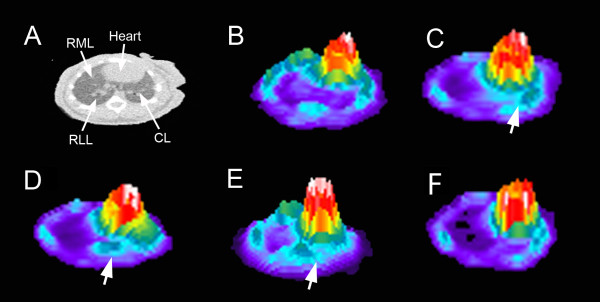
Intensity plots of FDG uptake in the cardiac lobe at various times after pneumonectomy. (A) A CT image of the transverse plane of the post-pneumonectomy lung identifying the location of the heart, right middle lobe (RML), right lower lobe (RLL), and cardiac lobe (CL). FDG-PET intensity profiles of a similar transverse plane on the day of surgery (B) and days 3 (C), 7 (D), 14 (E), and 21 (F) after pneumonectomy. Apparently increased uptake in the cardiac lobe is denoted (large arrow). Representative images at each time point are shown.
